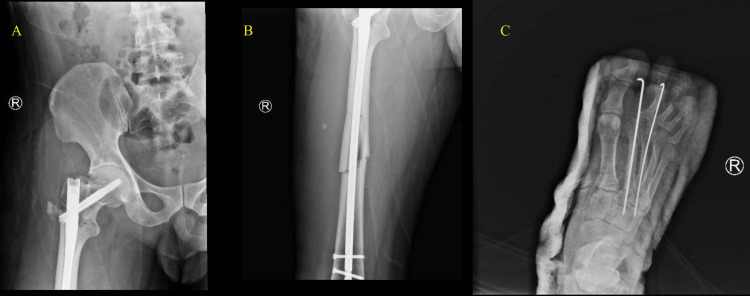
Immediate postoperative X-ray for the femur nail (A, B) and metatarsal K-wires (C)
